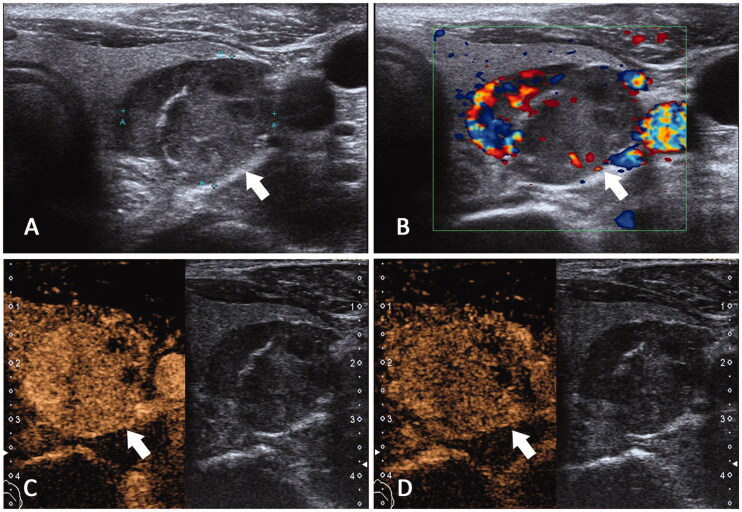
Ultrasonography feature of SHPT patient with high iPHT. A 55-year-old male diagnosed as SHPT for 8 years with high iPTH (3305pg/ml). (A) CUS detects a hypoecho lesion about 3.3 cm with calcification(white arrow). (B) Color Doppler showed the lesion has grade III blood supply. (C, D) In CEUS this lesion appeared to be hyper-enhanced in early phase and iso-enhanced in late phase.
